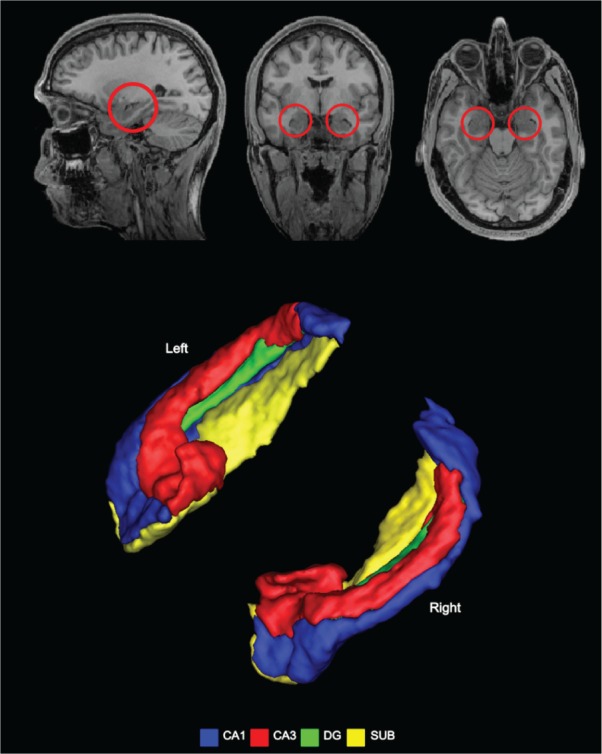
The human hippocampus. The top panel shows the hippocampi circled in red on sagittal (left), coronal (middle) and axial (right) views from a structural MRI brain scan. The hippocampus is composed of a number of subfields, CA1, CA2, CA3, which are adjoined by neighboring areas—the dentate gyrus (DG), the subiculum (SUB), presubiculum, parasubiculum, and entorhinal cortex—to form the extended hippocampal formation. Three-dimensional images of two example hippocampi are shown with some of the subregions indicated (from Bonnici and others 2012).
